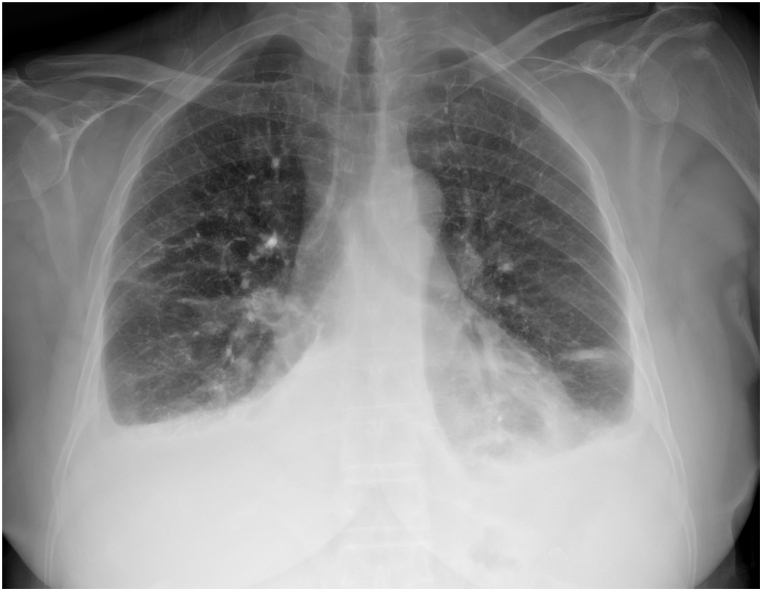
Chest radiograph on hospital day 14 showing interval radiologic improvement, with decreased bilateral opacities and stable pleural effusions.
